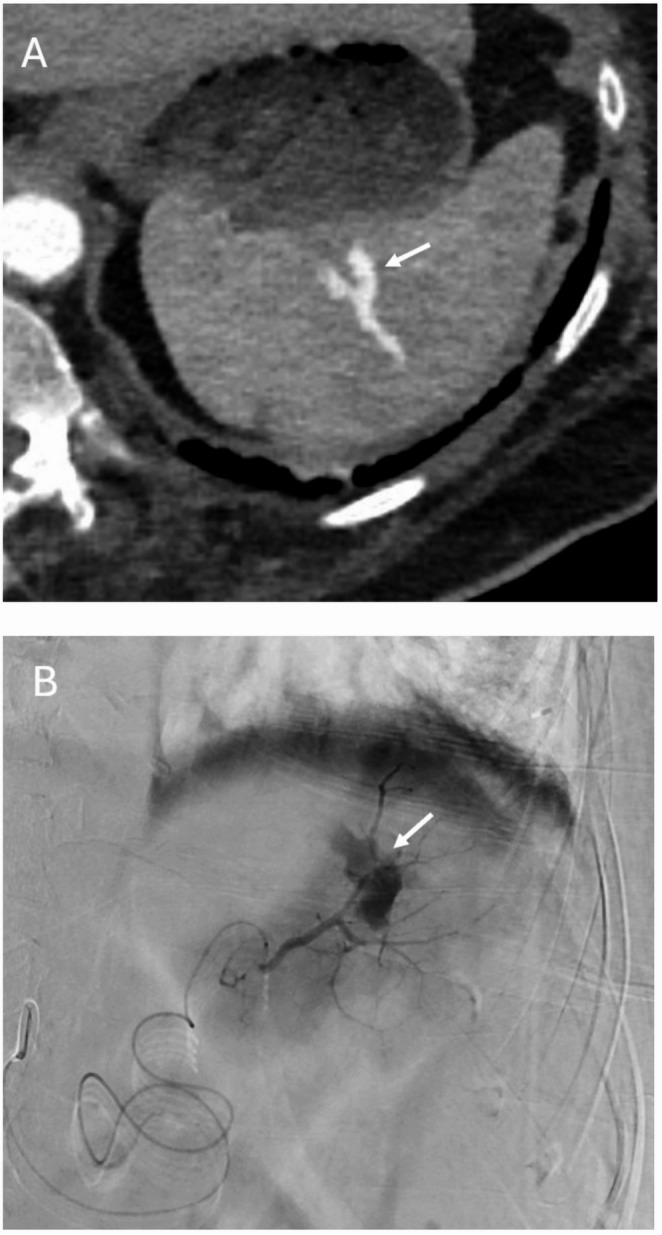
84-year-old female who fell down the stairs. Axial arterial phase CT (A) demonstrates an abnormal curvilinear contrast collection within the spleen (arrow), consistent with a pseudoaneurysm (AAST grade 4 injury) which was not mentioned in the original outside report. Subsequent angiography confirmed the presence of a traumatic splenic pseudoaneurysm (B, arrow) which was successfully treated with coil embolization (not shown)
